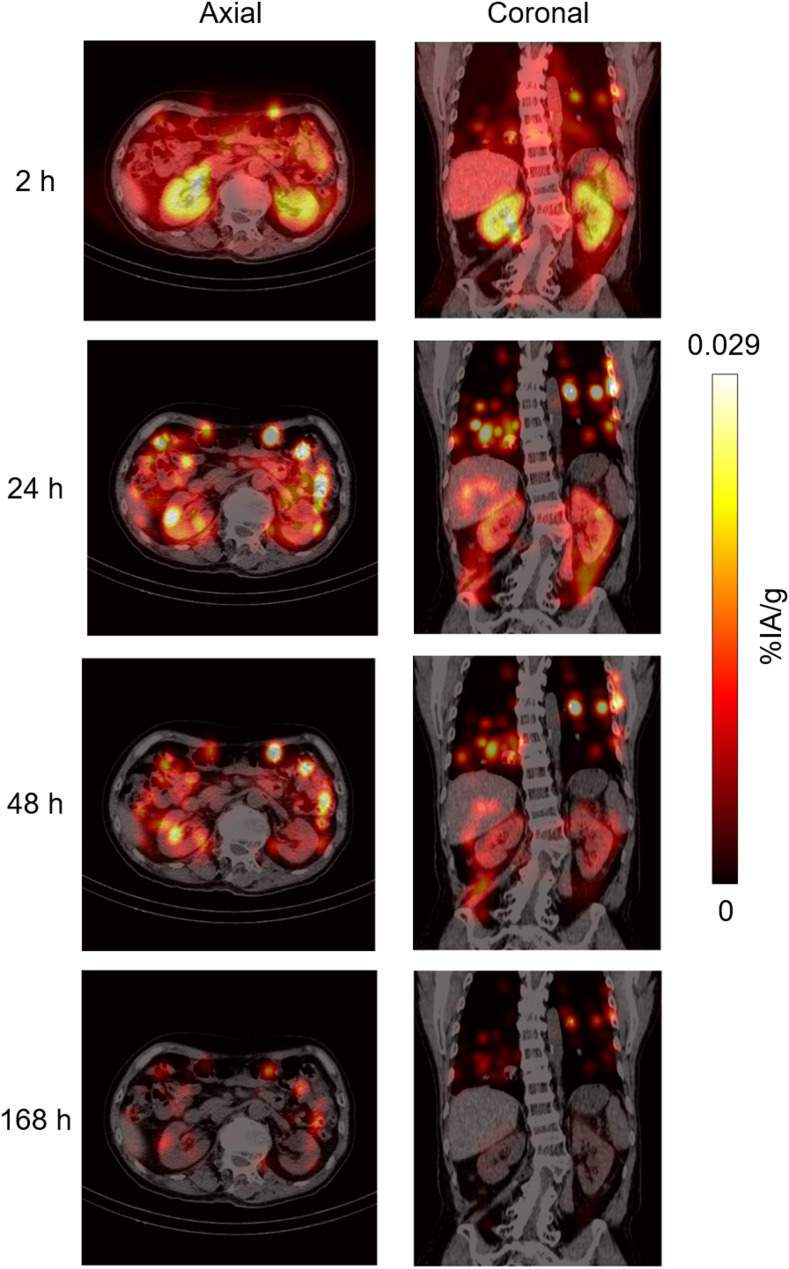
^177^Lu-PSMA-617 dosimetry imaging maximum intensity projection. PSMA, prostate-specific membrane antigen
